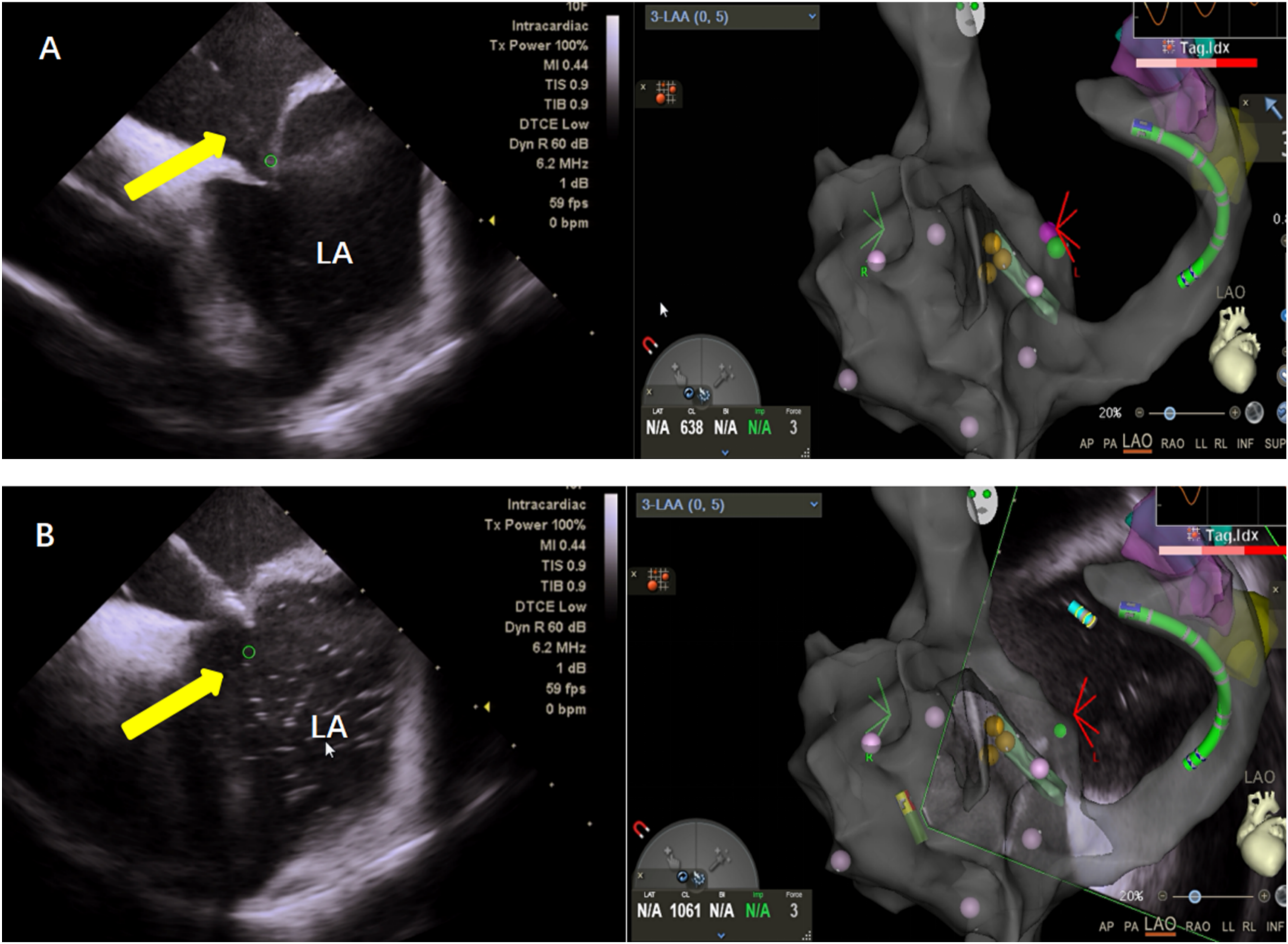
(A) Zero ray puncture: intracardiac ultrasound (left), showing puncture of the atrial septum under the guidance of the right atrial model and three-dimensional visualization of a patent foramen ovale (right). (B) Saline was injected into the puncture needle under direct visualization with intracardiac ultrasound (left), confirming successful puncture of the atrial septum.
